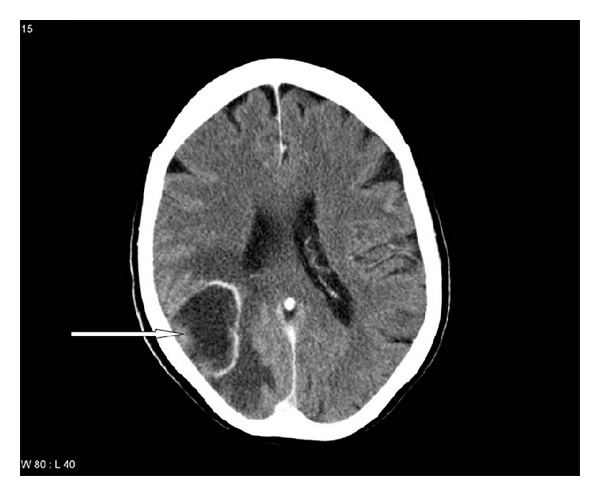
Contrast-enhanced computed tomography of the brain showing a 4.5 cm large, partially necrotic brain metastasis with oedema (overall 5 metastases were present).
